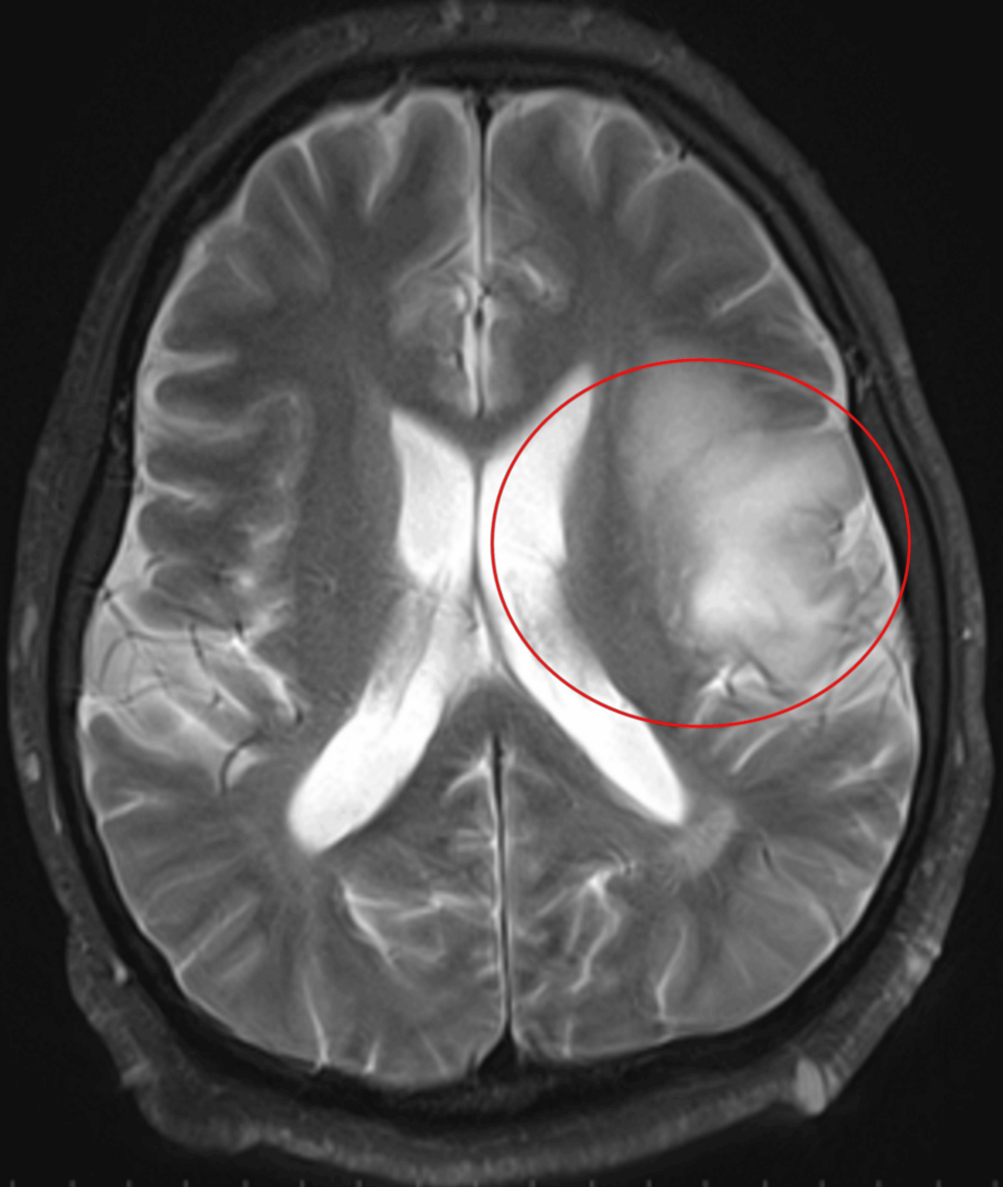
MRI revealed a high intensity area in the left insular gyrus
